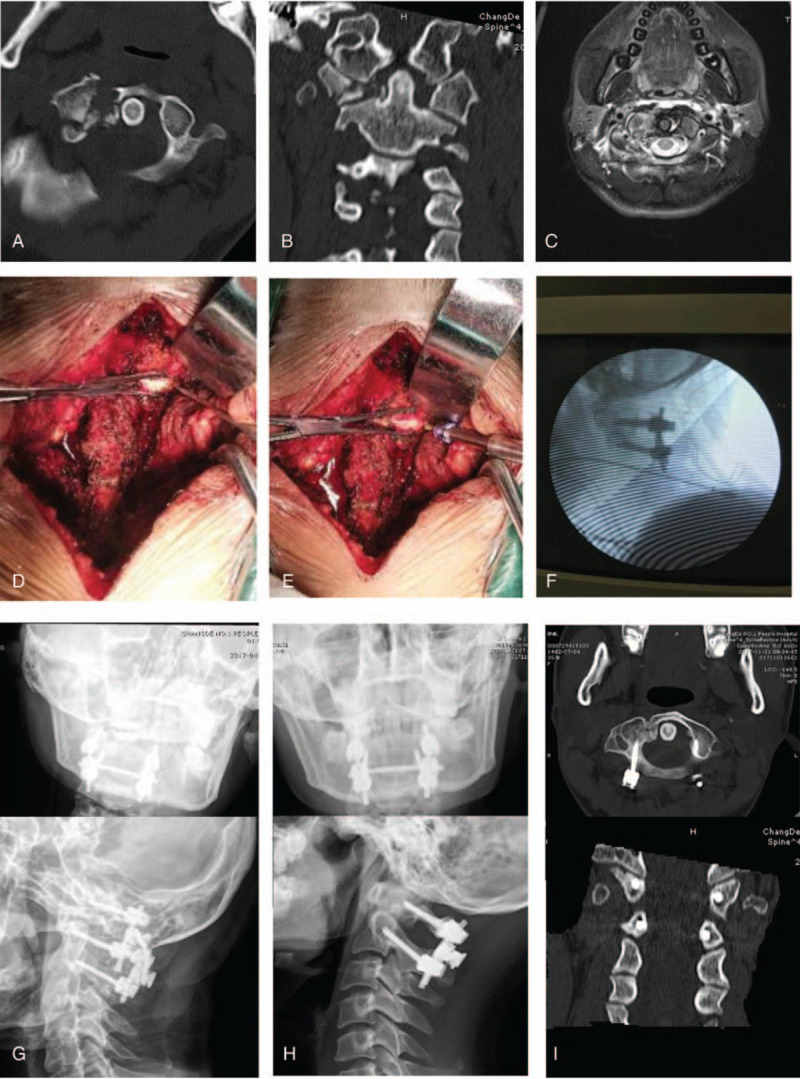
Pre-, intra-, and postoperative imaging of a 35-year-old female patient with Gehweiler classification type III atlas fracture. (A) and (B) Axial and coronal three-dimensional CT reconstruction images showed anterior and posterior arch fractures, and lateral mass burst fracture and displacement. (C) Axial magnetic resonance images showed partial transverse atlantal ligament rupture. (D) and (E) Intraoperative photographs showing the use of a large towel clamp to fix the lateral mass, then notching and screw insertion into the lateral mass of the atlas. (F) C-arm fluoroscopy was used to determine the position of the internal fixation. (G) and (H) Anteroposterior and lateral radiography showing fracture reduction and internal fixation positioning immediately postoperatively, and 3 months postoperatively. (I) Three-dimensional CT reconstruction at 6 months postoperatively showed fracture union.
